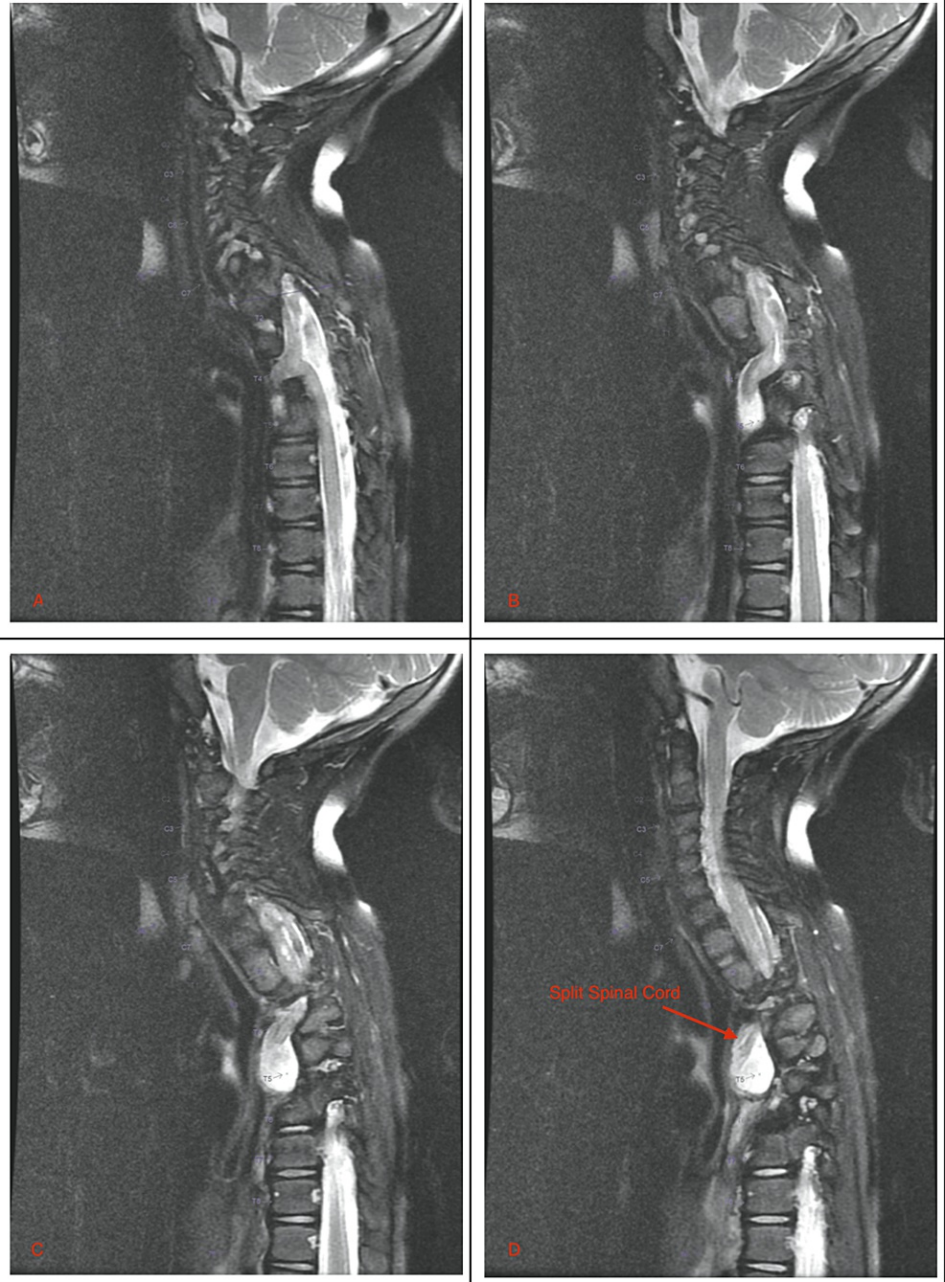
Sagittal cuts from T2-weighted MRI showing ventrally herniating myelomeningocele at T3-T4 containing CSF and spinal cord elements MRI: magnetic resonance imaging, CSF: cerebrospinal fluid
